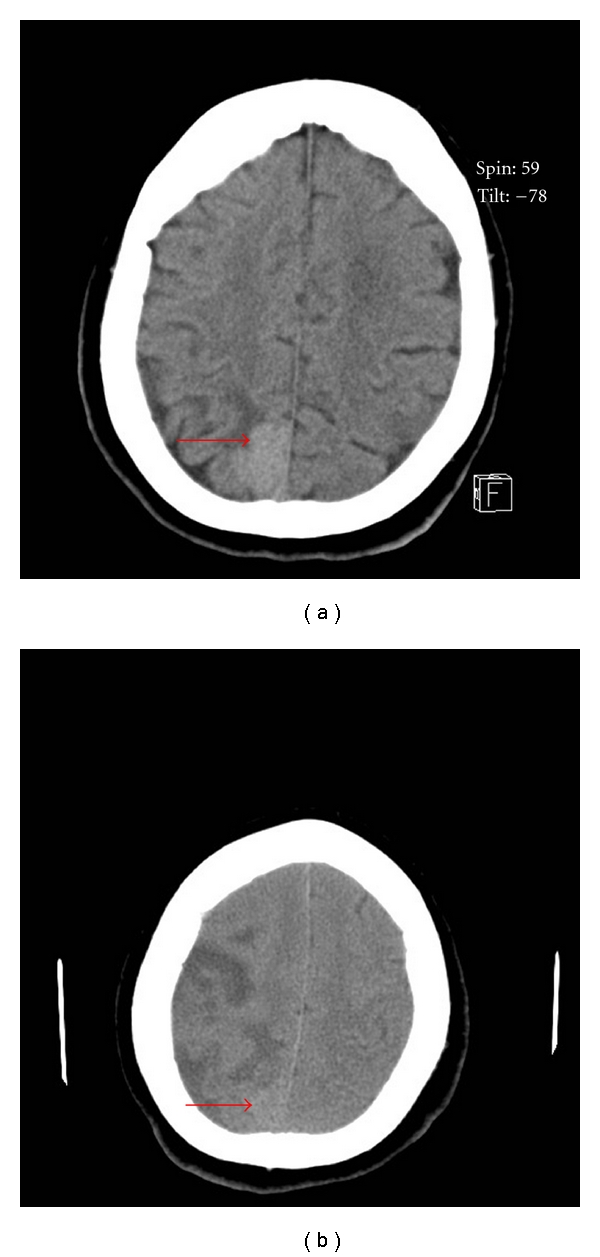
(a) Initial head CT showing parafalcine mass (arrow). (b) Head CT 36 hours after t-PA.
